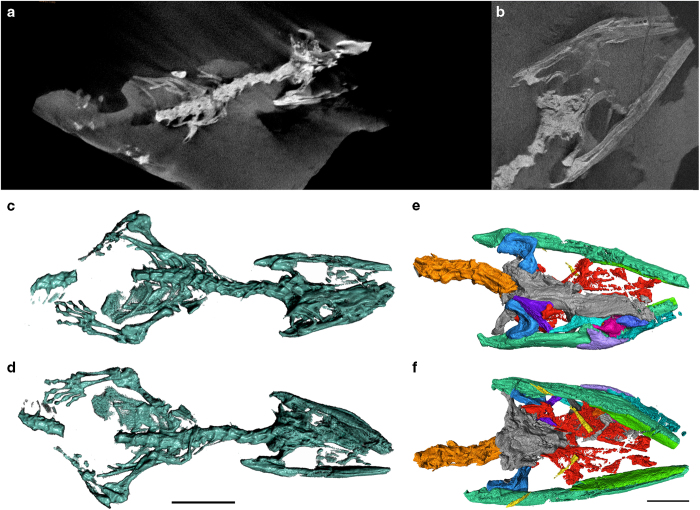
Segmentation procedure of the CT scan data of *Megachirella wachtleri*. (a) Sample slice of the whole-body scan of *Megachirella*. (b) Sample slice of the head-only scan of *Megachirella*. Each scan is composed of hundreds or thousands of slices that were subsequently used for individual segmentation procedures. (c) Whole skeleton after segmentation in dorsal view. (d) Whole skeleton after segmentation in ventral view. (e) Skull and mandibles after segmentation of individual components of the head in dorsal view. (f) Skull and mandibles after segmentation of individual components of the head in ventral view.
